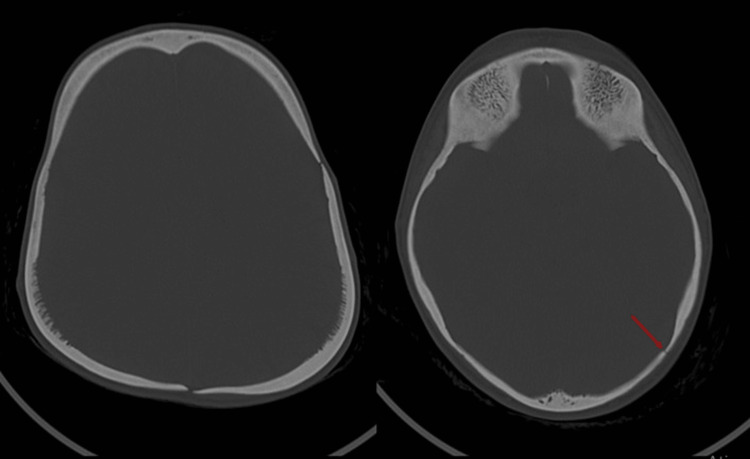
Axial CT view of the head showing heterogeneous bone density and sclerotic radiations of the parietal bones. Arrow indicates the regions of sclerotic thickening.
